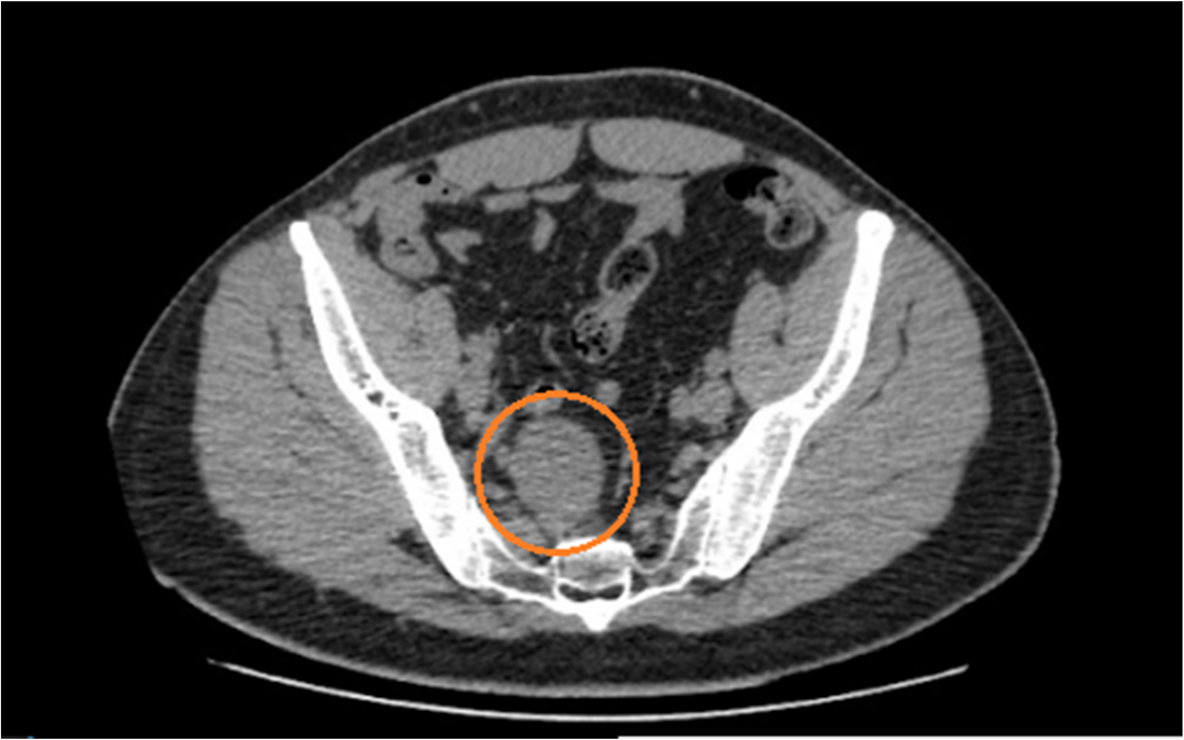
CT of the transversal plane of the presacral region. Note the schwannoma (red circle), with dimensions of 4.4 × 3.9 × 3.4 cm
